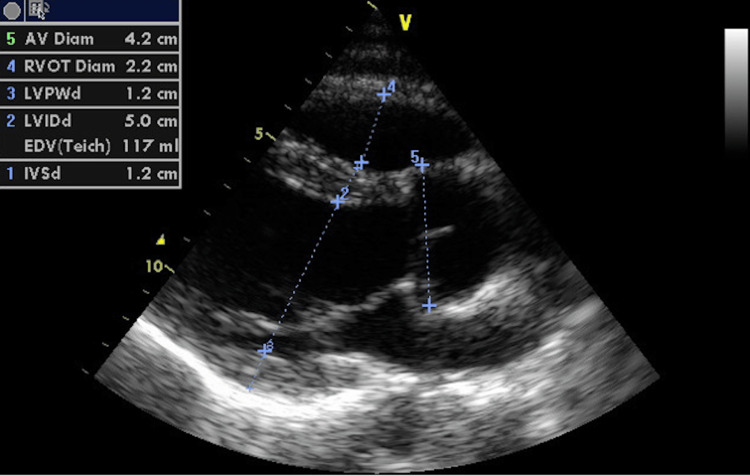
Echocardiogram: post-operative image of echocardiogram showing a non-dilated left ventricle with normal wall motion and systolic function, and no abnormalities
